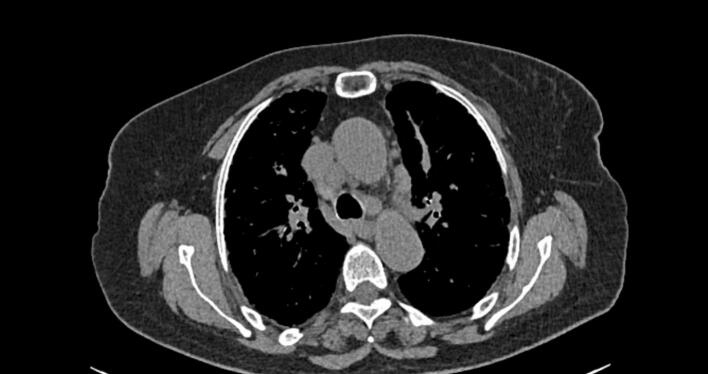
Computed tomography of the thorax revealing a diffuse interstitial pneumonia pattern with mediastinal lymphadenopathies.
